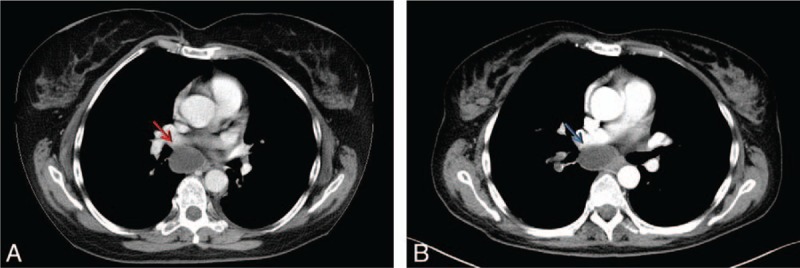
Enhanced-CT axial sections. (A) In 2016, the subcarinal lymphnode (red arrow) changed from hypodense to isodense and appeared reduced in size. (B) One year later, the same lesion (blue arrow) appear stable.
